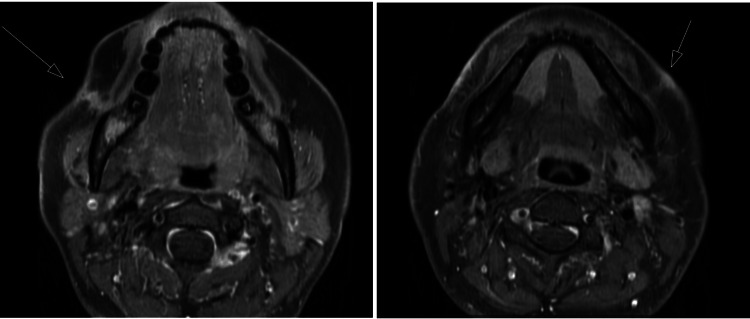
Initial MRI findings (A) A tract of edema and enhancement that extends to the skin surface, where there is mild puckering, thickening, and enhancement. (B) A linear tract of subcutaneous soft tissue edema and enhancement leading to the overlying skin surface, with mild skin thickening and enhancement but without puckering.
